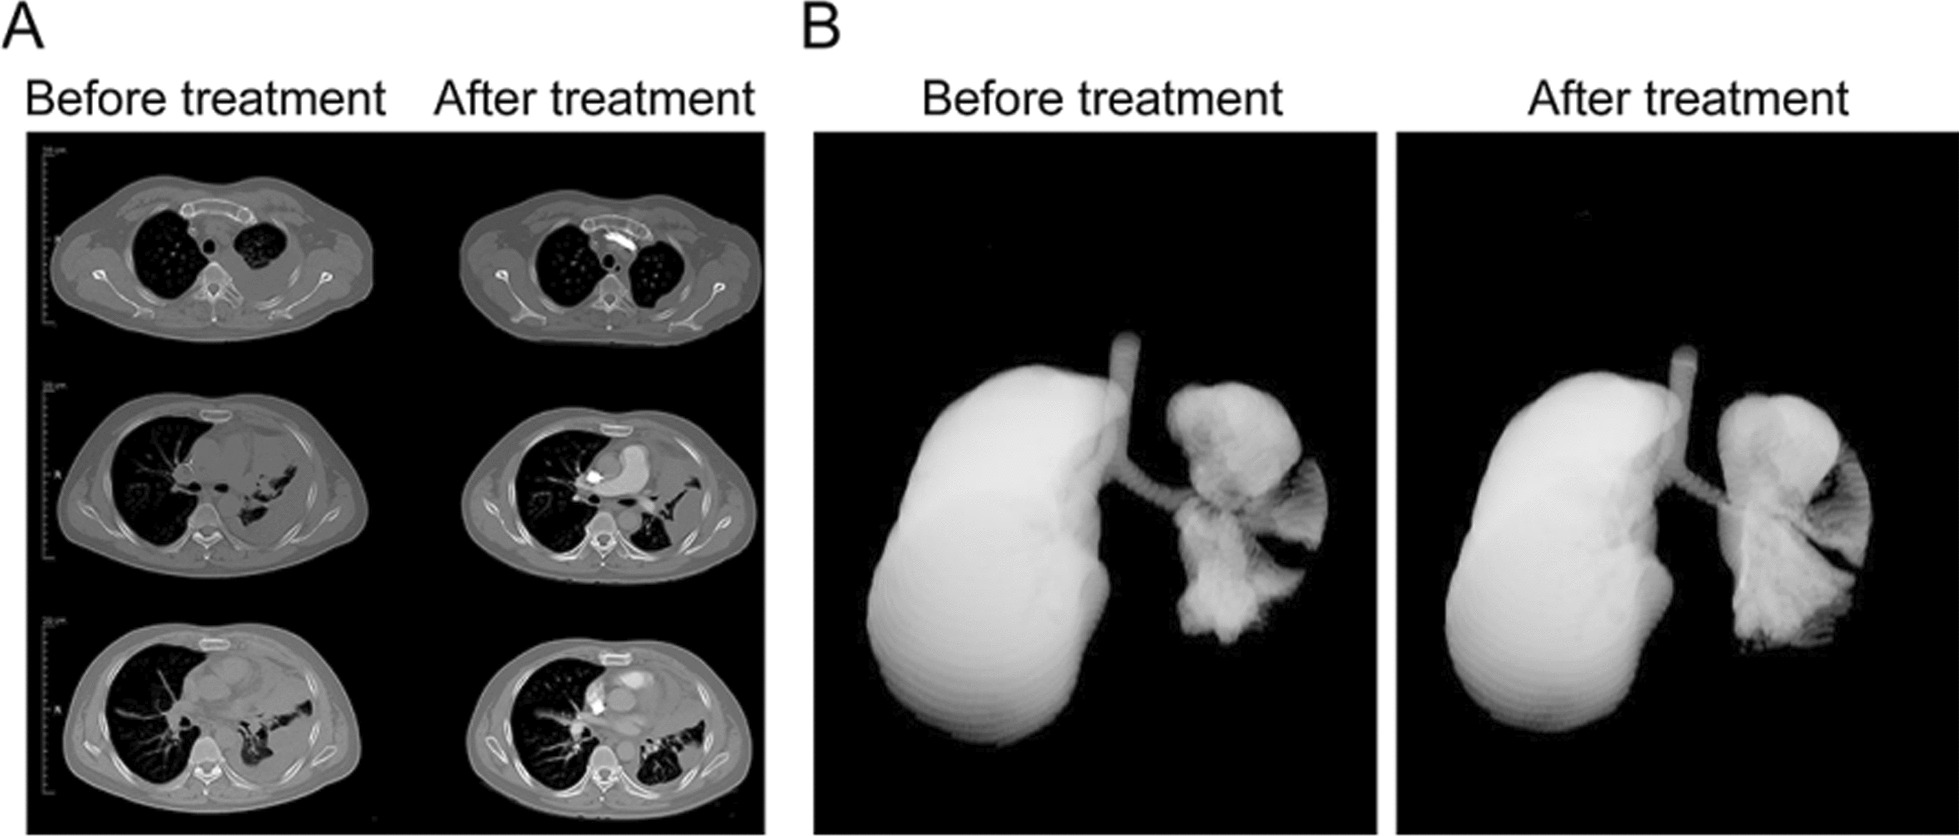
Therapeutic effects of the drugs selected by D~Sense assay in patient. A CT scan images before and after the combination therapy of Gemcitabine and Lobaplatin. B The 3D reconstituted lung airspace images before and after the combination therapy of Gemcitabine and Lobaplatin. showing a 16% increase of volume after therapy
